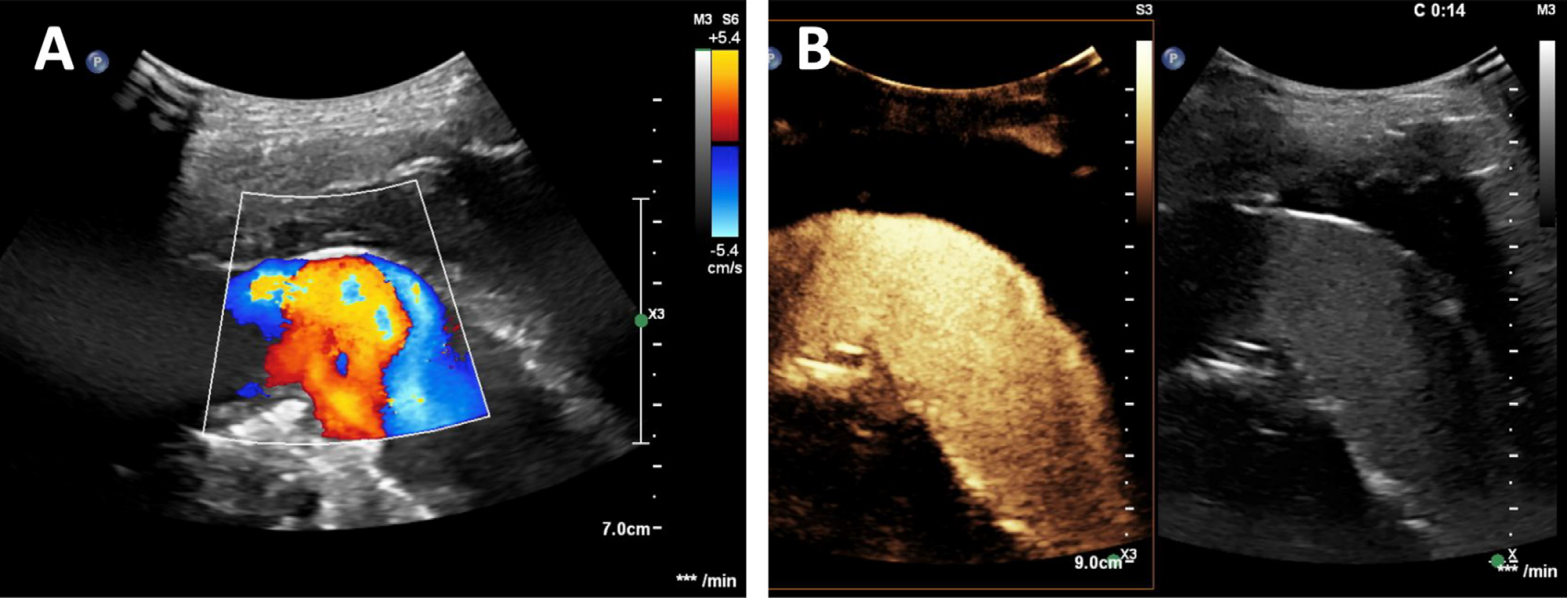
Color-coded Doppler ultrasound (A) shows flow in the stented aneurysm but no flow in the excluded aneurysm sac. Contrast-enhanced ultrasound (B) also shows no perfusion of the excluded aneurysm sack.
